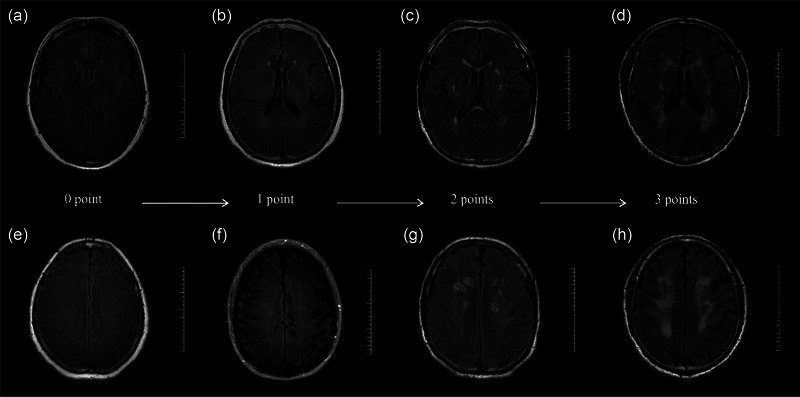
Magnetic resonance imaging (MRI) imaging of cerebral small vessel disease (CSVD) patients with different severity of white matter hyperintensity (WMH) in T2FLAIR. Periventricular WMH (PWMH): (A) (0 point), (B) (1 point), (C) (2 points), and (D) (3 points); deep WMH (DWMH): (E) (0 point), (F) (1 point), (G) (2 points), and (H) (3 points).
